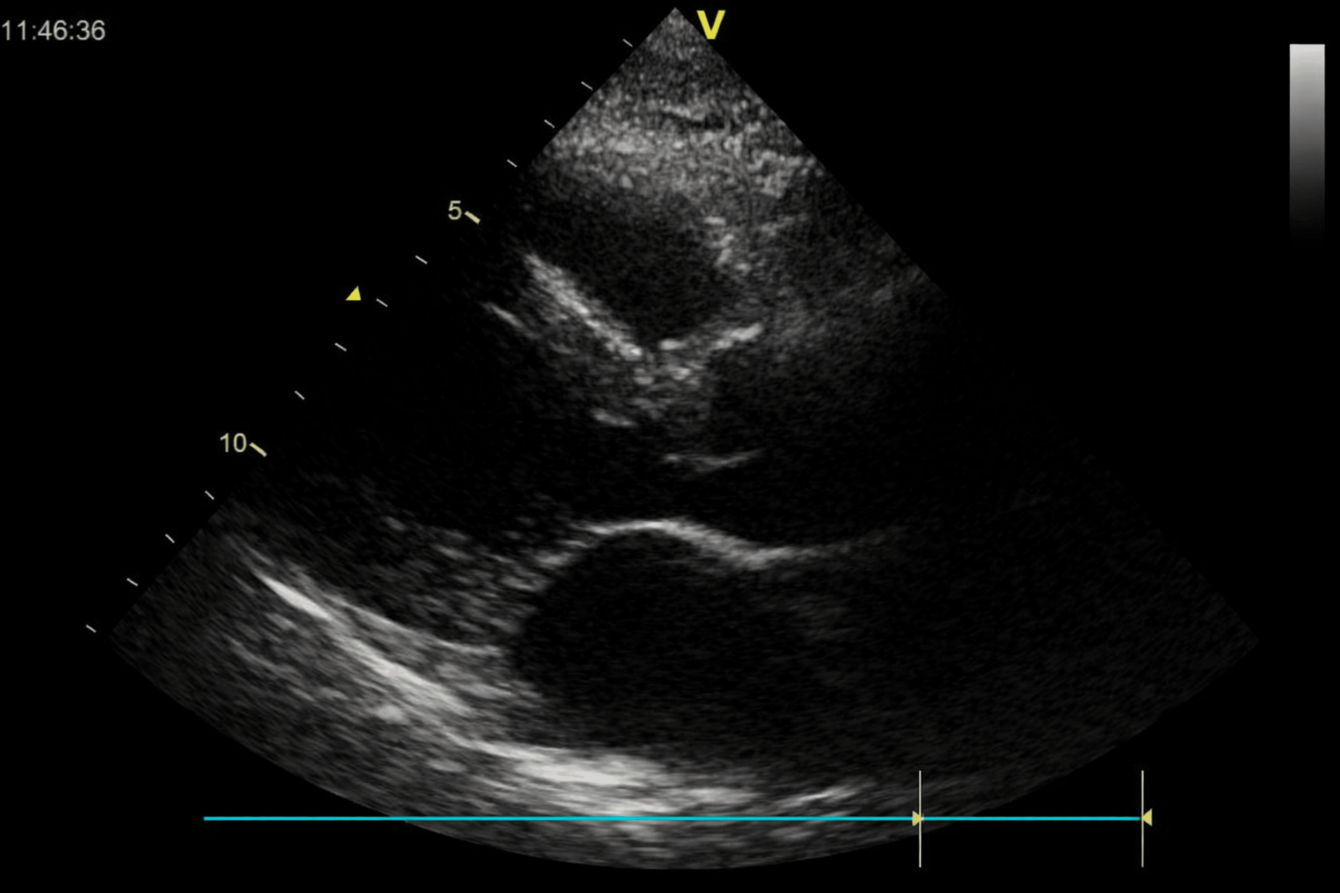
Transthoracic echocardiography findings Parasternal long-axis view demonstrating preserved left ventricular systolic function with mild concentric hypertrophy and no significant chamber dilation.
